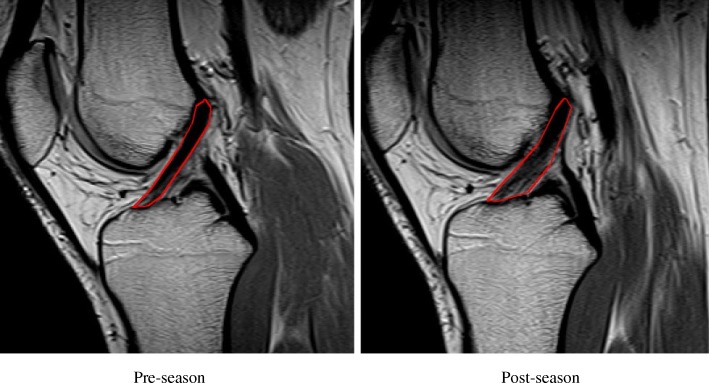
MRI Image of Same Knee at Pre and Postseason
